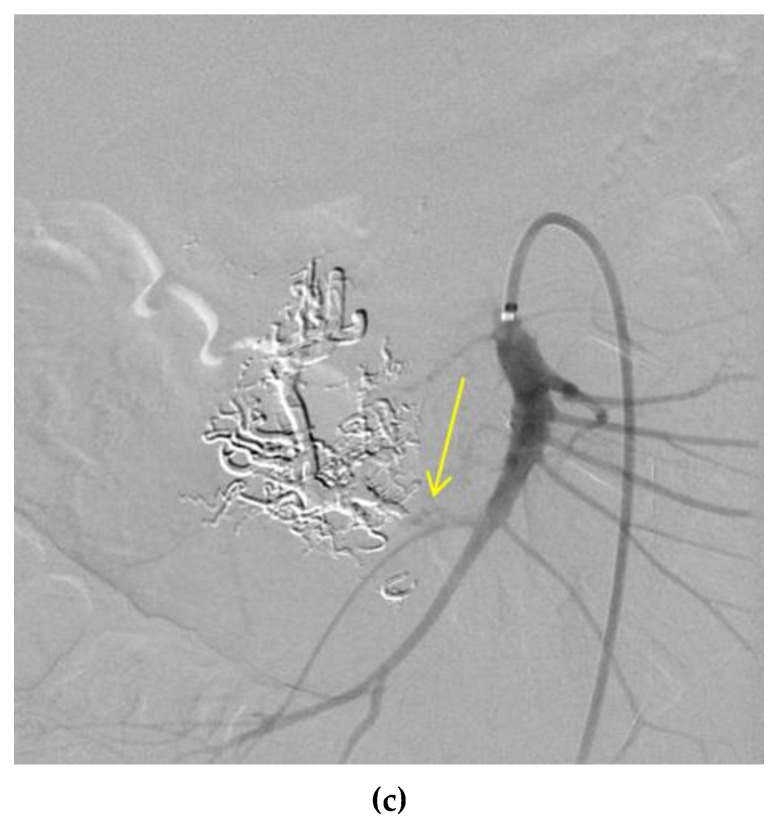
Embolization of a pancreatic AVM. Onyx is used to occlude a nidus with feeder arteries from both the gastroduodenal and superior mesenteric (SMA) arteries. Draining vessels include the superior mesenteric vein (SMV) and portal veins. (a) The image shows the presence of a spongiform nidus, with a “blush” of arteriole-venule connections, indicative of a Cho–Do type IIIa or Yakes type II AVM. (b) The image from a common hepatic artery digital subtraction arteriogram (DSA) shows the occlusion of gastroduodenal artery feeders; and (c) The image from an SMA DSA shows occlusion of SMA feeders. The liquid embolic agent has hardened and is dense (subtracted in these images) due to the addition of tantalum powder for radiographic visualization.
